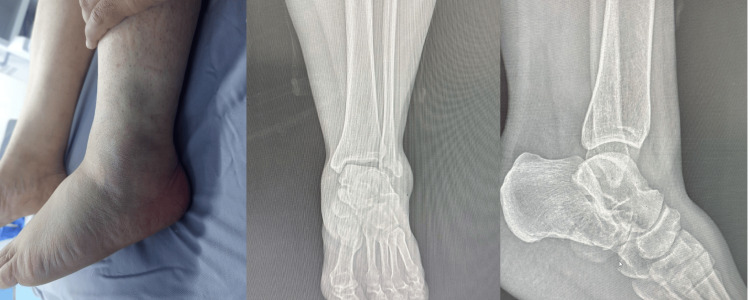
Ankle swelling with lateral ecchymosis; anteroposterior and lateral ankle radiographs. Clinical photograph showing swelling of the ankle and foot with lateral ankle ecchymosis (left). Anteroposterior ankle radiograph demonstrating a distal fibular tip fracture with associated talar tilt (middle). Lateral ankle radiograph showing the “double-density” sign, indicative of overlap of the lateral calcaneal wall (right). However, diagnosing calcaneal fracture-dislocation on plain radiographs can be difficult and requires a high index of suspicion, with CT imaging needed for confirmation.
